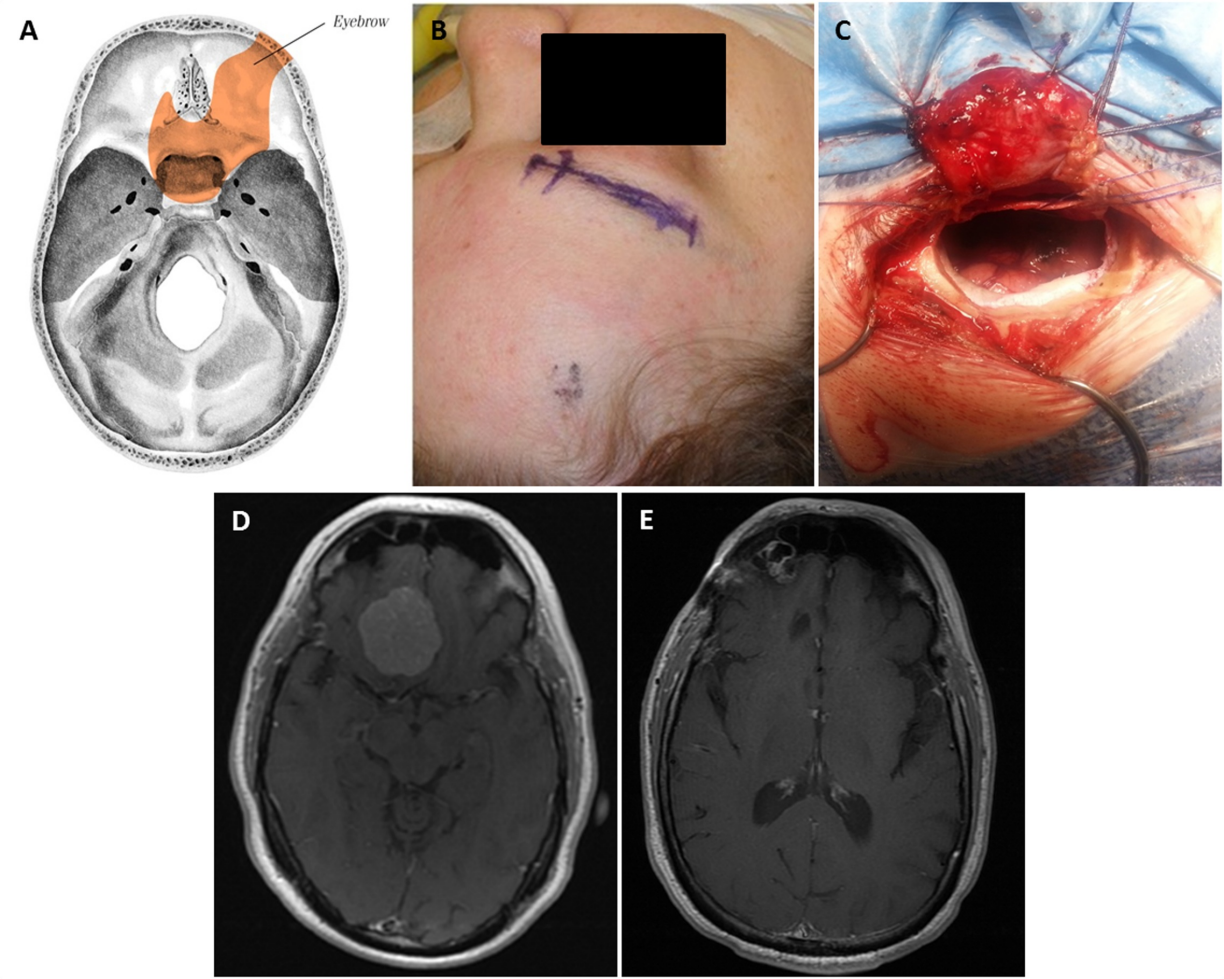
Supraorbital “Eyebrow” Keyhole Approach The skull base regions accessible with this approach (A). Planned incision (B) and craniotomy (C). Preoperative post-contrast axial T1 MRI shows right planum sphenoidale/anterior skull base meningioma (D). Postoperative axial MRI (E).
